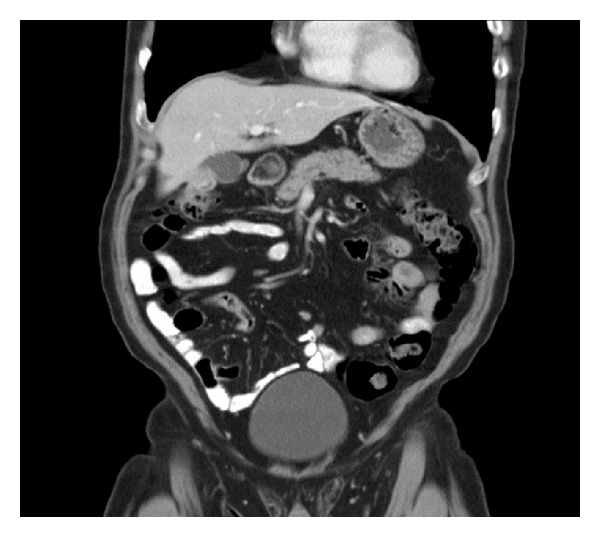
CT scan showing ovoid mass in gallbladder.
